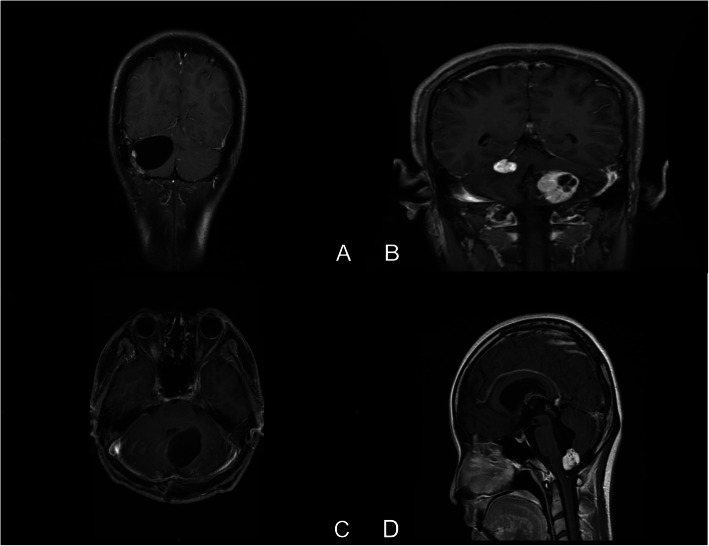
Postcontrast MRI of cerebellar HGB and peritumoral cyst (a and c), solid cerebellar tumor and intratumoral cyst (b), and HGB in the medulla oblongata (d)
